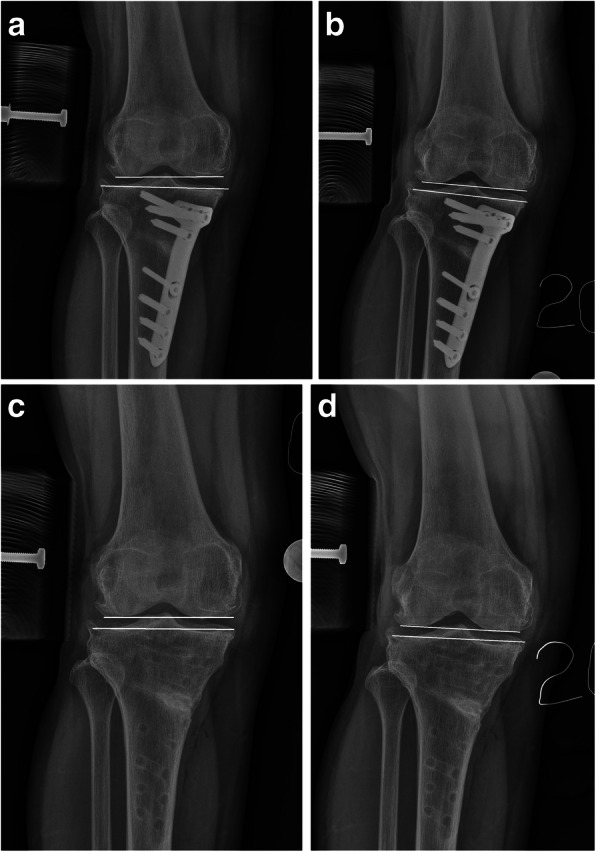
The joint line convergence angle was measured using radiographs. The angle between two lines is joint line convergence angle. a A valgus force of 40 N was exerted at extension before plate removal. b A valgus force of 40 N was exerted at a flexion position of 20° before plate removal. c A valgus force of 40 N was exerted at extension after plate removal. d A valgus force of 40 N was exerted at a flexion position of 20° after plate removal
